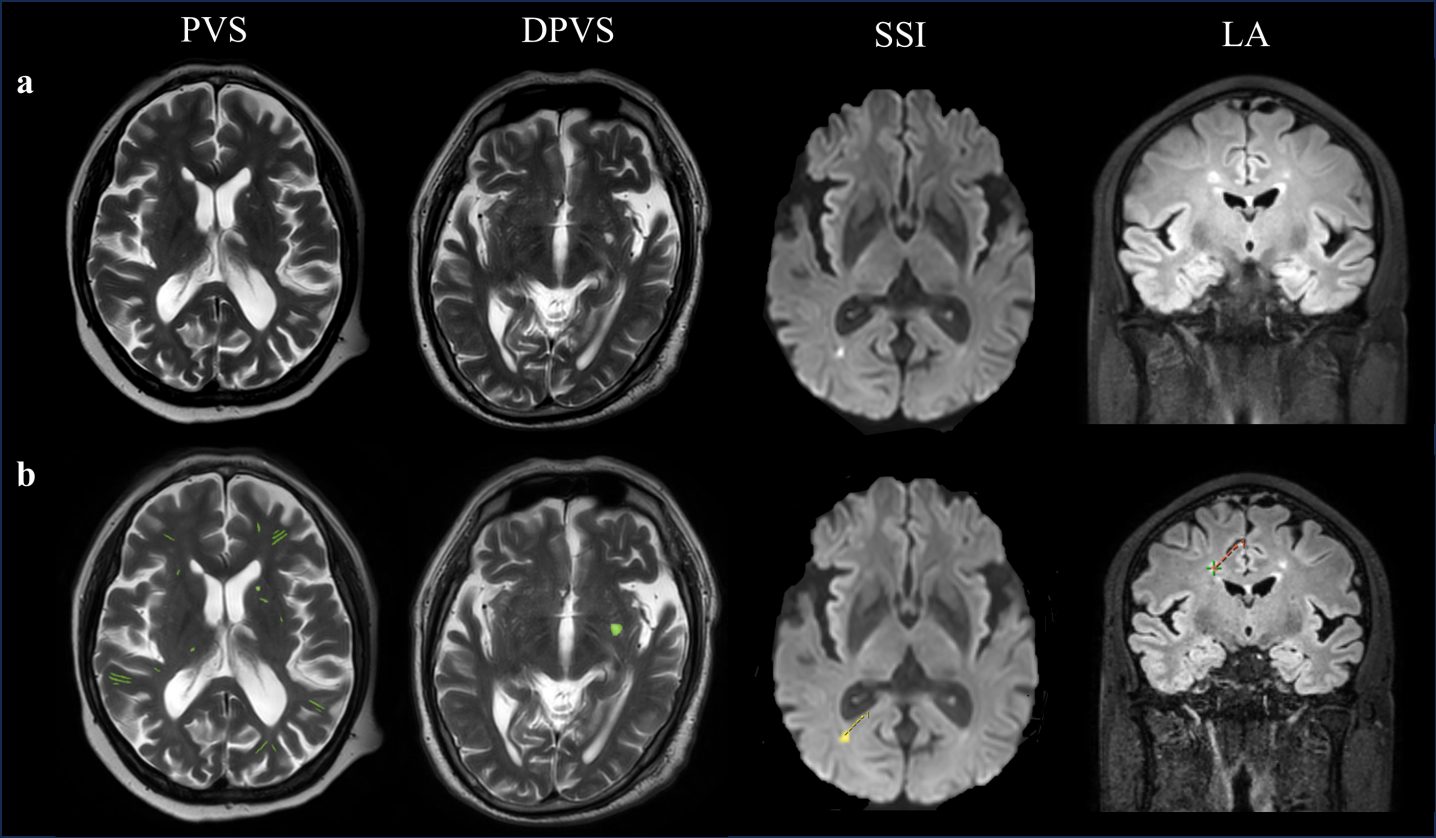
Quantitative steps of CSVD Imaging Features. (a) Original MRI images. (b) The analysis interface of uAI Research Portal.
